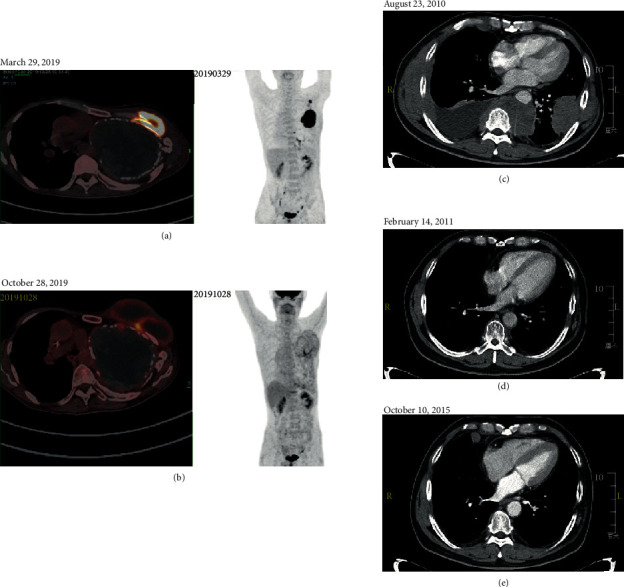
Imaging assessment of patients with artificial pneumothorax-unrelated pyothorax-associated lymphoma (PAL). Positron Emission tomography/computed tomography (PET/CT) scan in patient 1 (a-b) and contrast-enhanced chest CT  scan in patient 2 (c–e) were performed at the indicated time points.
